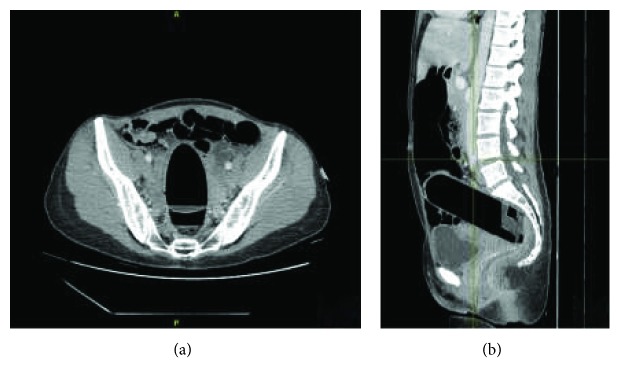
The visualization of the foreign body by CT: axial view (a) and sagittal view (b).
